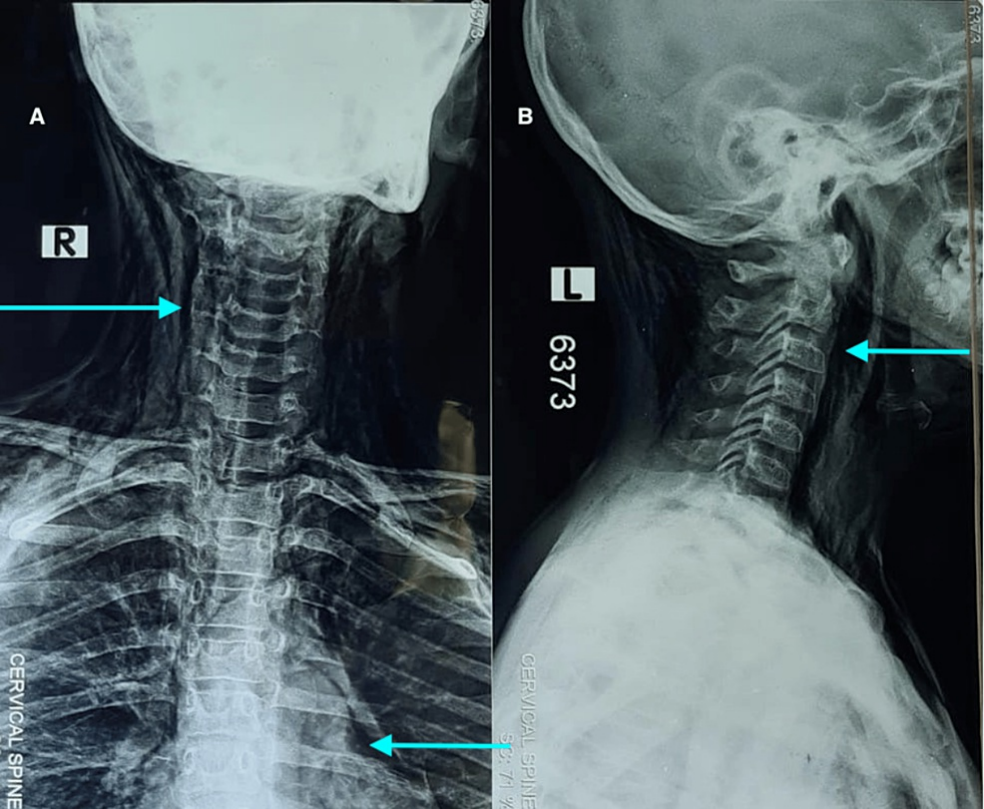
Radiograph of the neck AP (A) and lateral (B) Note that air within the soft tissue planes of the neck (upper arrow in A), pneumomediastinum (lower arrow in A), and parapharyngeal air (arrow in B)
